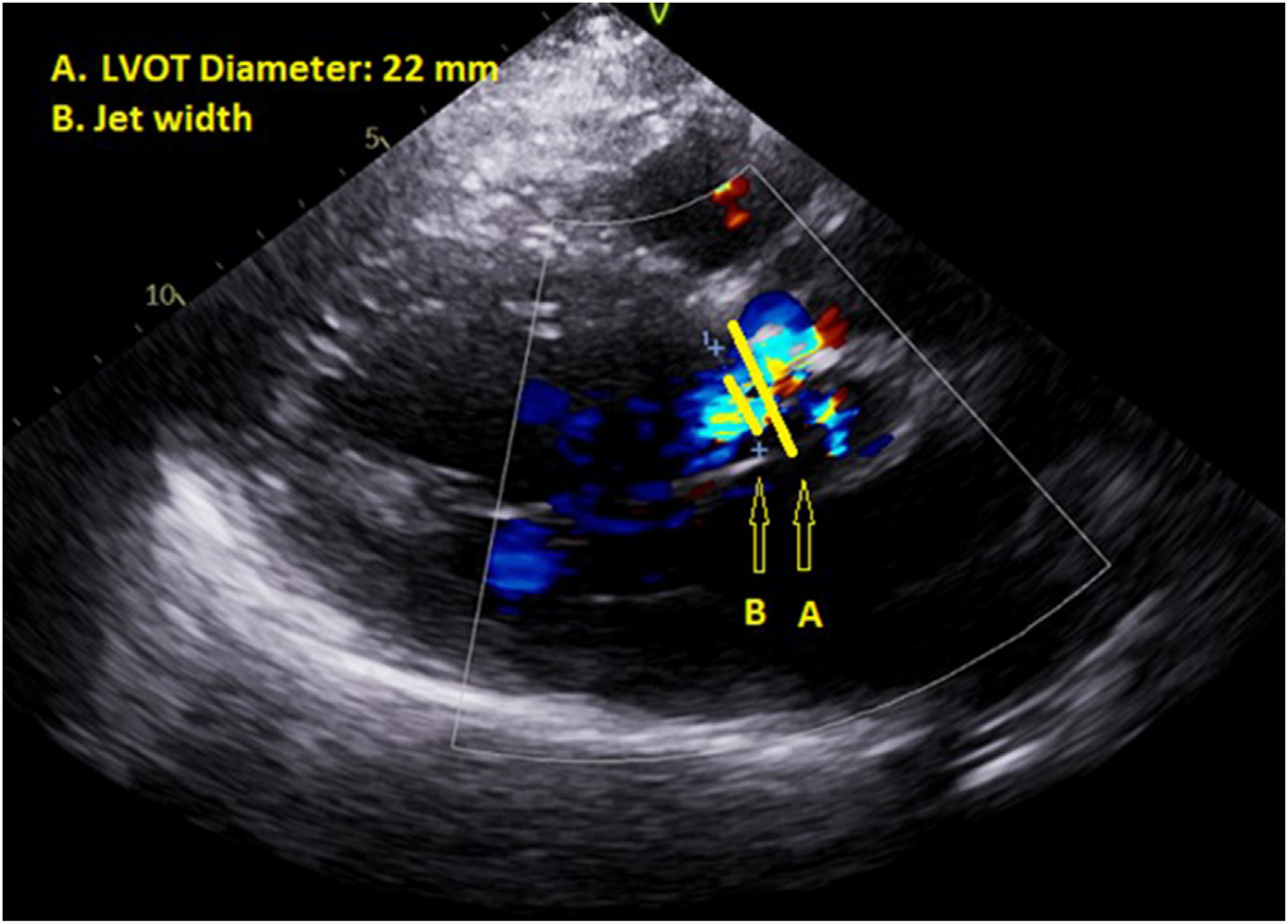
Two-dimensional TTE, parasternal long-axis diastolic view with color-flow Doppler, demonstrates a dilated left ventricular cavity, increased left ventricular wall thickness, and a bioprosthetic AV with moderate paravalvular regurgitation (jet width [B] to LVOT [A] ratio of 40%). *LVOT*, Left ventricular outflow tract.
